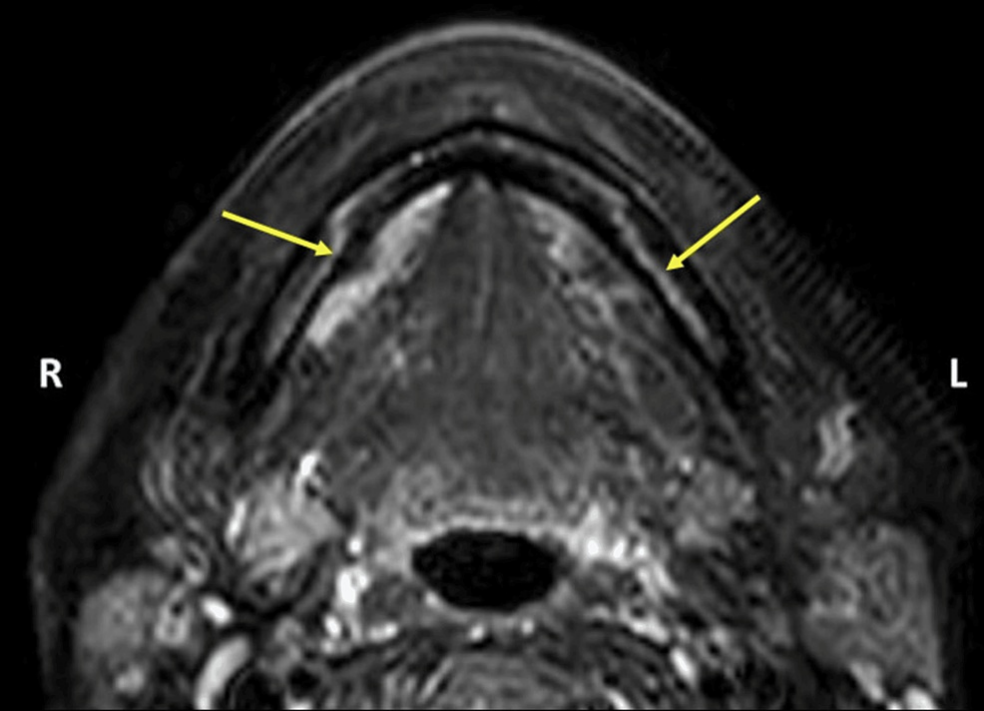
T2-weighted axial section showing the course of the inferior alveolar nerve in the mandible (yellow arrows)
